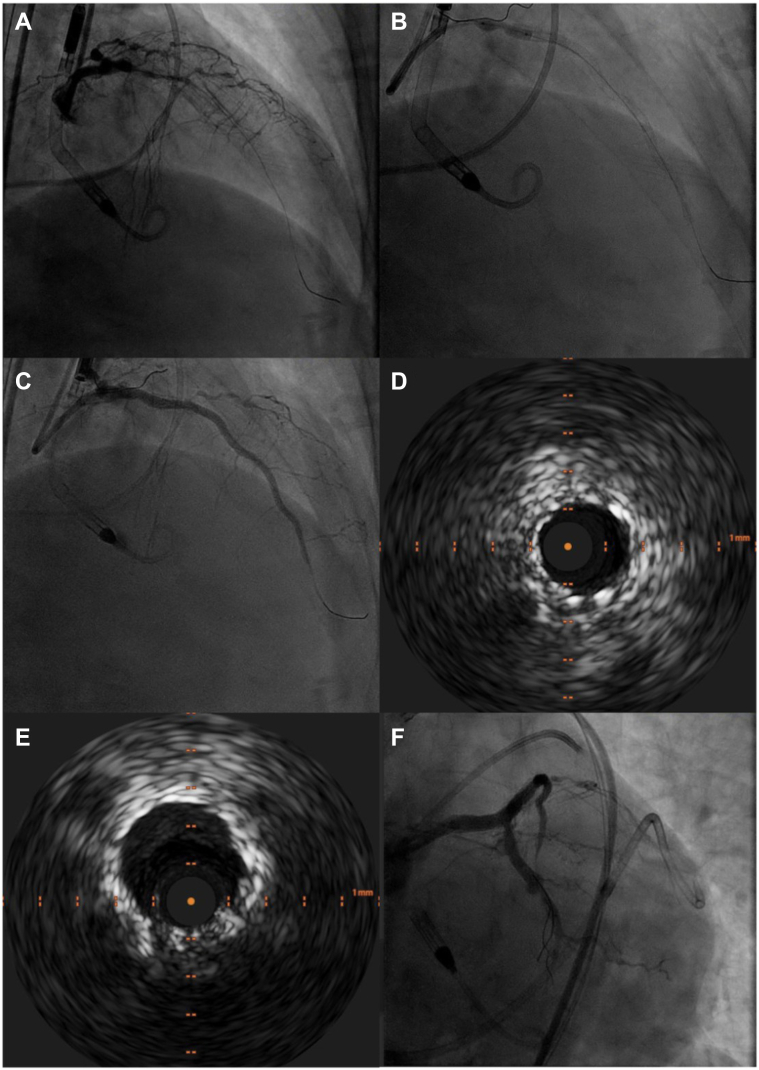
Management of coronary artery perforation in proximal left anterior descending (LAD) artery. (A) Baseline angiogram showing lesion in proximal LAD. (B) Balloon inflation resulting in coronary artery perforation. (C) Covered stent placement to manage the perforation. (D) Intravascular ultrasound (IVUS) visualization of the covered stent before dilation. (E) IVUS-guided postdilation of the covered stent. (F) Final angiogram demonstrating resolution of perforation after IVUS-guided postdilation of the covered stent.
